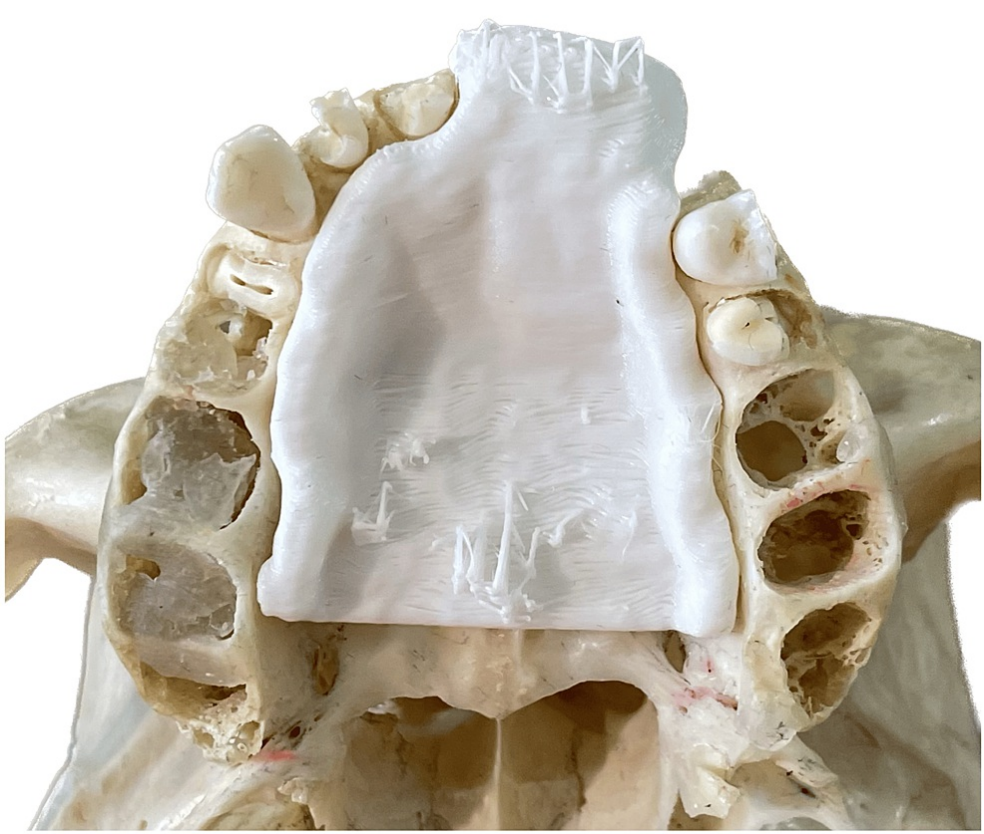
The 3D-printed obturator, designed from 360-degree protocol CBCT data, placed on the skull. CBCT: cone beam computed tomography.
